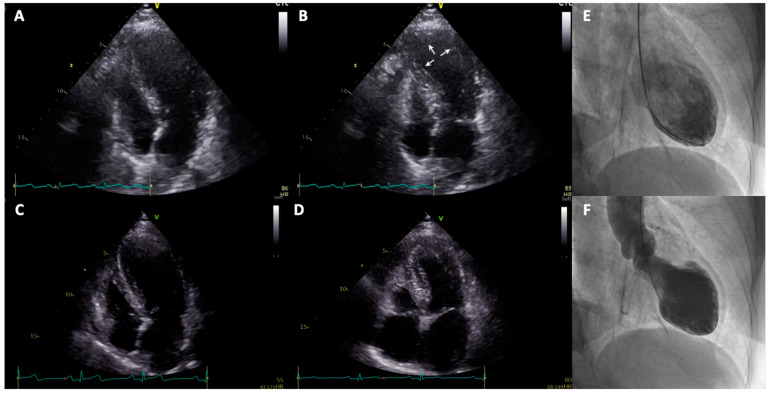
Apical 4-chamber view: in telediastole (A), in telesystole (B) showing apical ballooning (see arrows) in TTS on day 1; in telediastole (C), in telesystole (D) showing normal apical contractility on day 6. Left ventriculography (RAO 30°) in telediastole (E), in telesystole (F) on day 1 showing apical ballooning and hypercontractility of basal segments resembling typical Japanese octopus pot. RAO: right anterior oblique.
